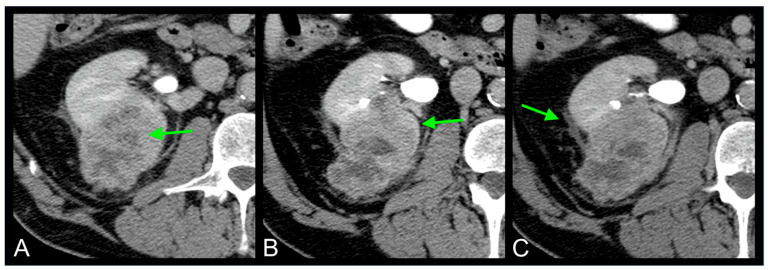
Axial CT images during excretory phase showing radiogenomic features of ccRCC with VSX1-positive expression: extensive tumor necrosis [arrow in (A)], collecting system invasion [arrow in (B)], and perinephric fat stranding [arrow in (C)].
